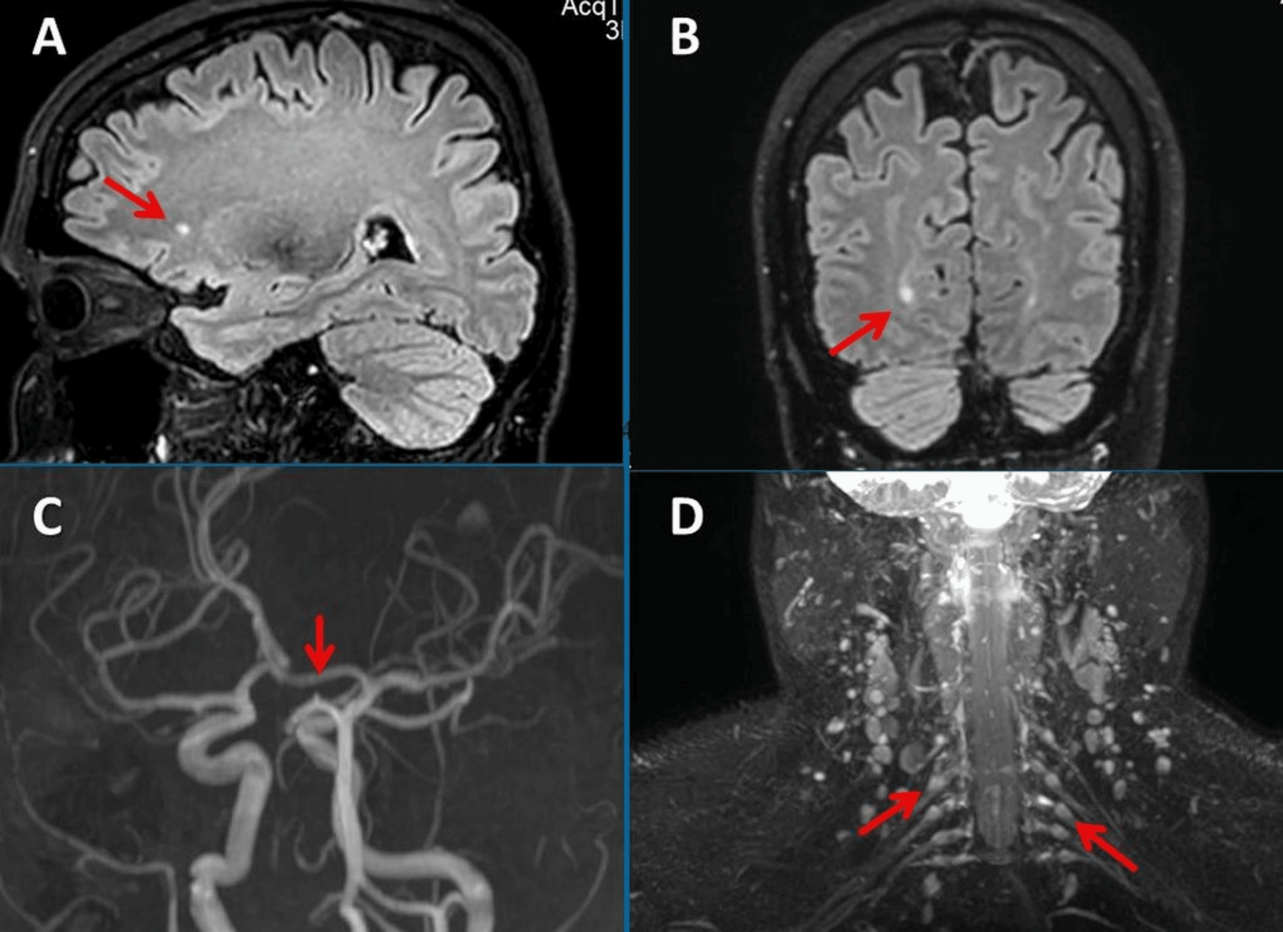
Cerebral MRI in January 2024 shows spot-like gliosis in the right frontal white matter on FLAIR (panels A and B). Time-of-flight MRA shows a small aneurysm in the P1 segment of the right posterior cerebral artery (panel C). Nerve MRI shows prominent dorsal root ganglia of the inferior cervical nerve roots, suggestive of ganglionitis (panel D) MRI, magnetic resonance imaging; FLAIR, fluid-attenuated inversion recovery; MRA, magnetic resonance angiography.
